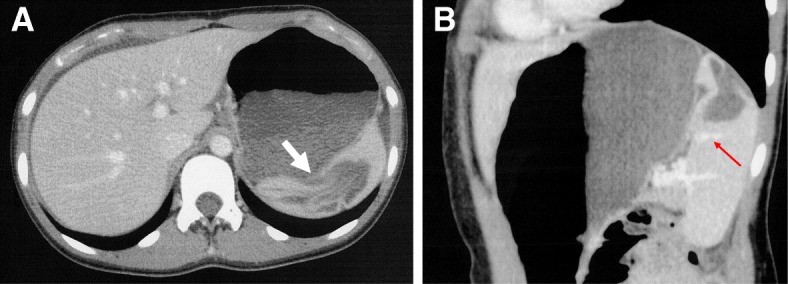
a Transverse contrast-enhanced CT images demonstrated a large, collapsed splenic cyst communicating with the peritoneal cavity through the ruptured cyst wall (white arrow), and no neoplastic nodules nor abnormal calcifications were detected. b Sagittal contrast-enhanced CT images demonstrated a ruptured cyst with a branch of splenic vessels in the splenic parenchyma near the border of the collapsed cyst at the upper pole of the spleen (red arrow)
